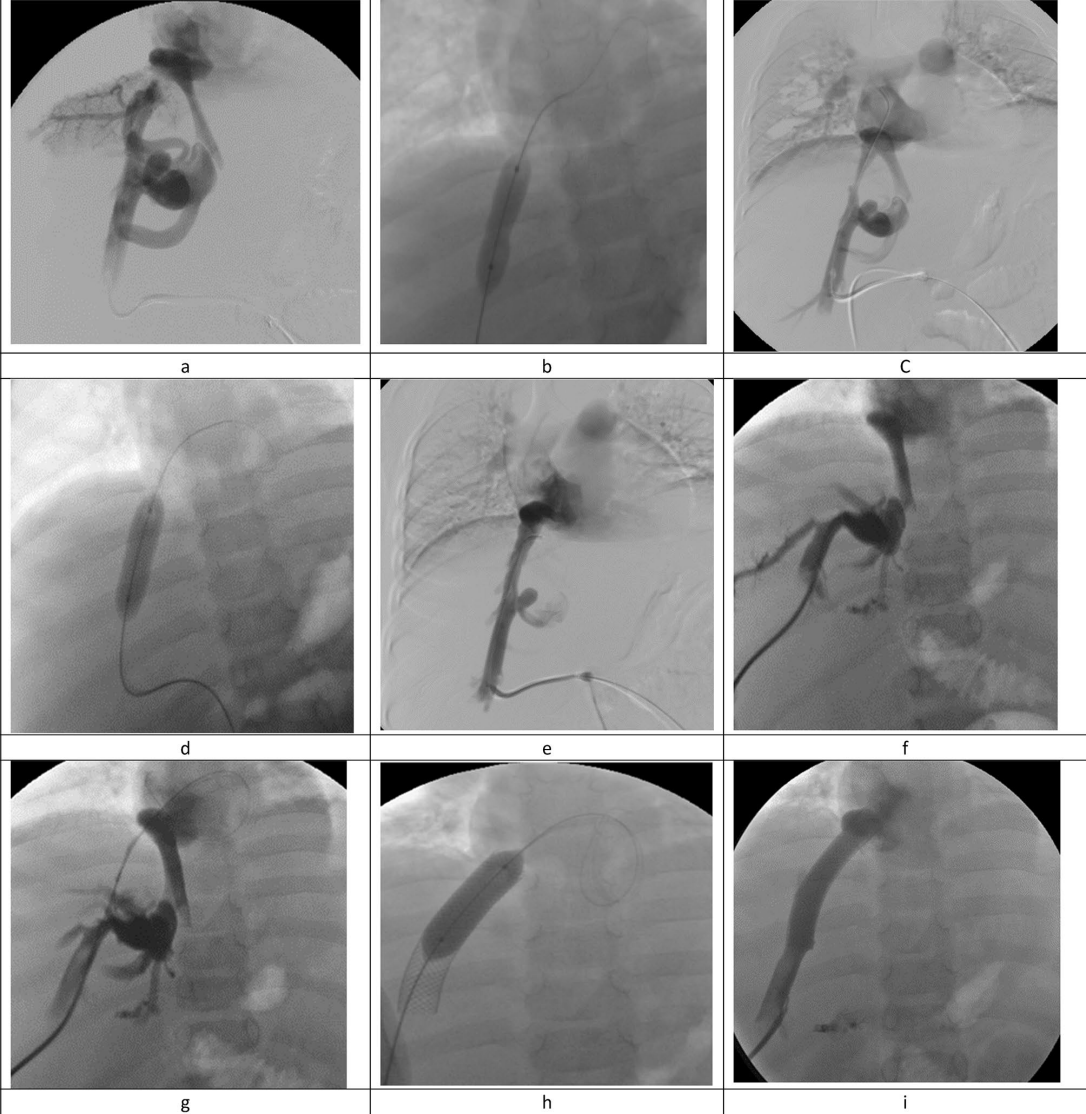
Angiography images of a 15-year-old female patients with FVLM: factor V Leiden mutation (FVLM) with obstruction of the right hepatic vein for 6 months. Transhepatic venography revealed short segment obstruction involving right HV ostium with multiple dilated vascular collaterals (a). Balloon dilatation was performed (b), venography revealed residual stenosis and persistence of collaterals (c). Repeat dilatation was performed (d) and control venography revealed good recanalization of the HV with disappearance of vascular collaterals and without residual stenosis (e). Patient presented with recurrent symptoms 10 months following HV angioplasty, and color Doppler ultrasonography revealed thrombosis of the previously treated right HV. Angiography revealed progressive thrombosis of the treated HV compared to the primary intervention with vascular collaterals (f). The obstruction was bypassed using a transhepatic approach (g) followed by stent insertion (h). Final venography following stent dilatation revealed free flow of contrast agent across the stent with disappearance of intrahepatic collaterals (j).
